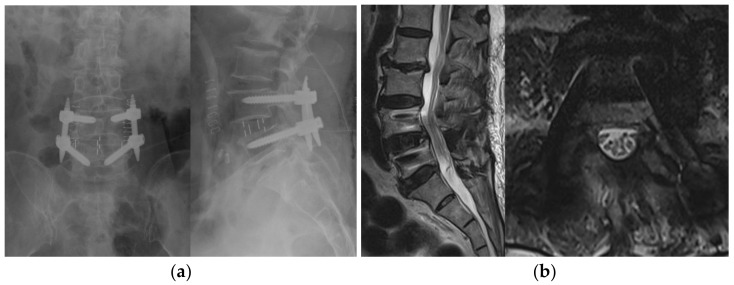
(a) A postoperative, simple radiographic image after BESS with OLIF. (b) The postoperative MR image reveals the complete decompression of SCCS at L4–5. BESS, biportal endoscopic spinal surgery; OLIF, oblique lumbar interbody fusion; SCCS, severe central canal stenosis.
